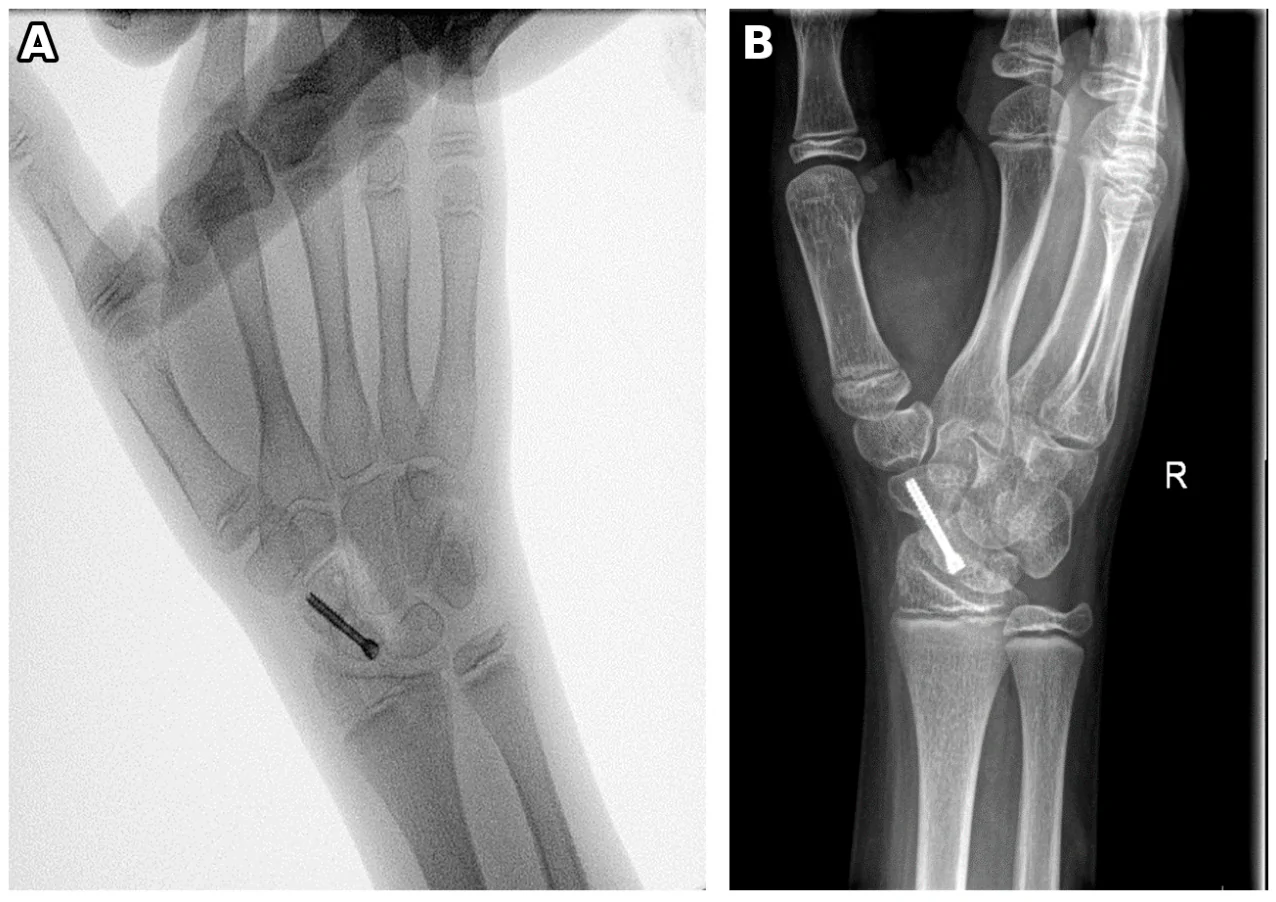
Fixation and early healing. (A) Immediate postoperative posteroanterior radiograph demonstrating the 2.4 mm headless compression screw and restored scaphoid alignment. (B) Posteroanterior radiograph obtained at two months demonstrating progressing union. Corresponding lateral views are provided in Supplementary Figure S5.
